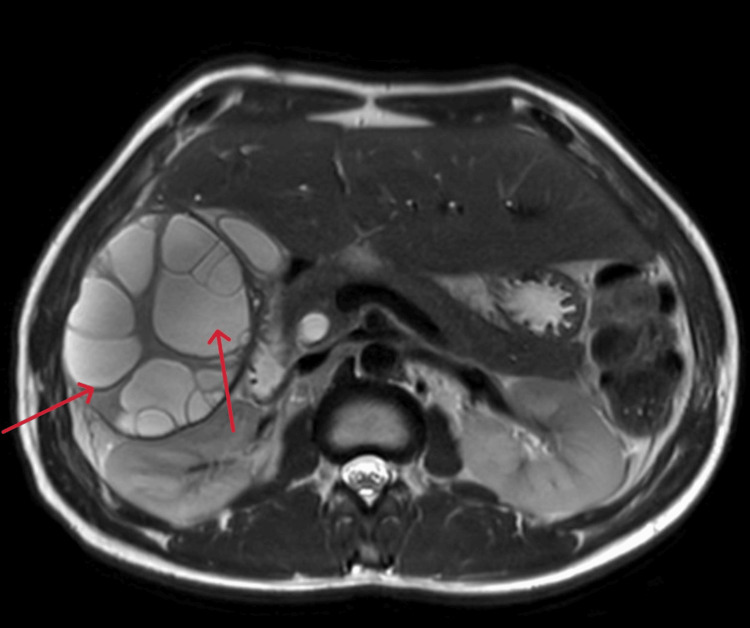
Axial MRI of the liver demonstrating hydatid cyst Axial MRI image of the liver showing a large hydatid cyst involving the right hepatic lobe. Multiple peripherally arranged daughter cysts are clearly visible within the main cystic lesion. MRI, magnetic resonance imaging
